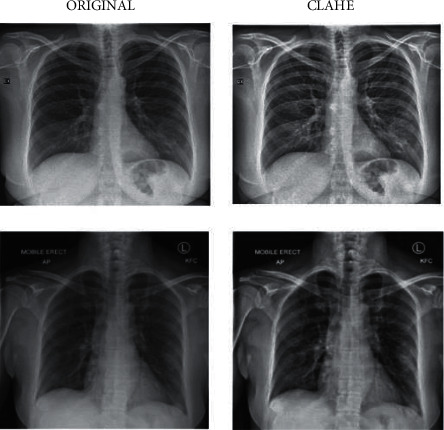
Original CT image versus CLAHE-processed CT image [145].
